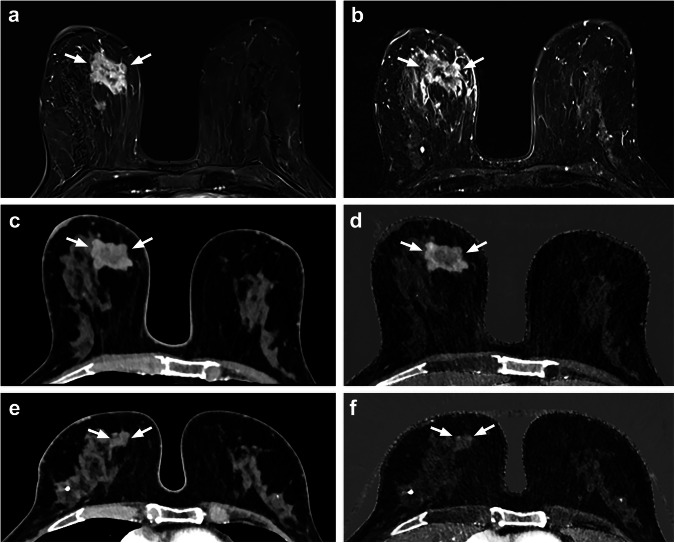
Patient with right-sided breast tumor (arrow) of no special type, G2, ER+/PR+/HER2-, visualized in breast MRI at initial diagnosis with T1w-subtraction after contrast administration (a) and fat-saturated T2w sequence (b). Photon-counting detector computed tomography with monoenergetic reconstruction (c, e) and iodine map (d, f) shows the tumor at initial tumor staging before NAST (c, d) and with a marked decline of tumor size after NAST (e, f). ER, Estrogen receptor; HER2, Human epidermal growth factor receptor; MRI, Magnetic resonance imaging; NAST, Neoadjuvant systemic therapy; PR, Progesterone receptor
